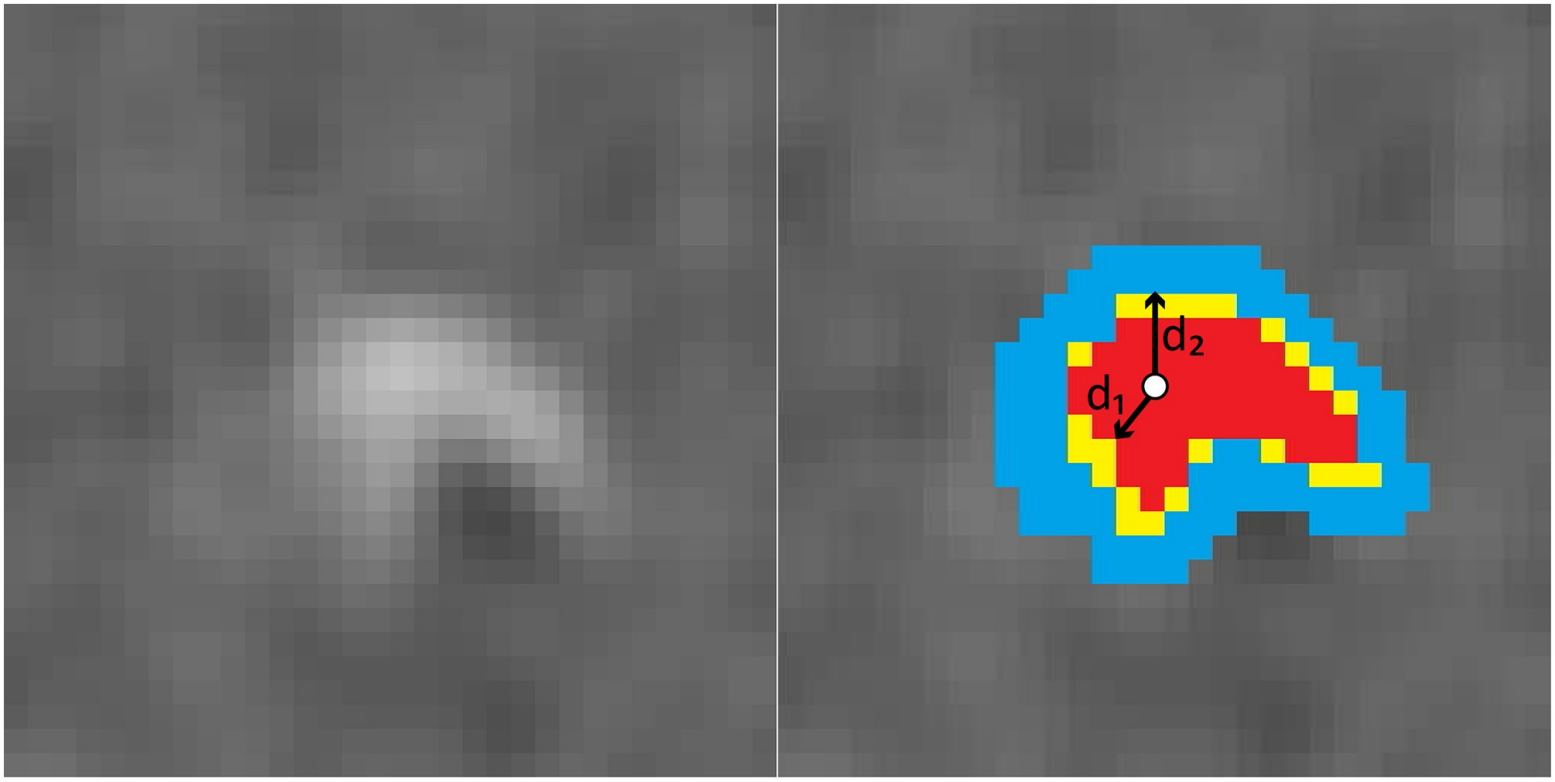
CAC segmentation using PVC-CS: Left: CT image containing calcified lesion; Right: Initial calcification volume obtained by intensity value thresholding at 130 HU (red); Refined extended calcification segmentation obtained by intensity value thresholding at 90 HU filtered with the distance transform (yellow); CAC background (blue).
